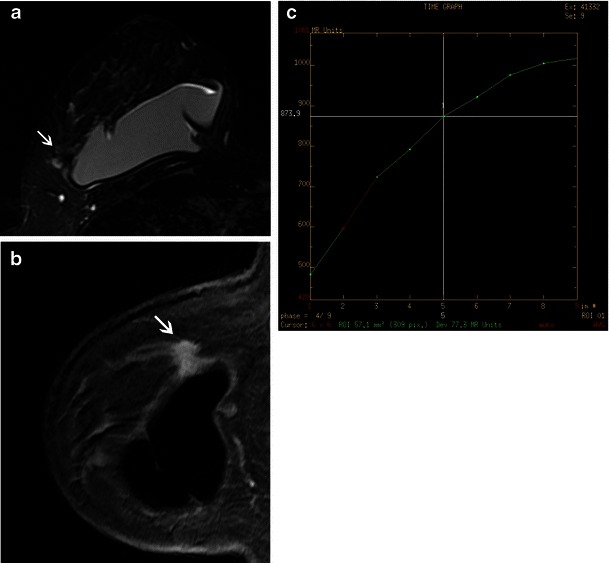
A 45-year-old woman with ILC. a and b MR images from right breast, respectively, T2 with fat suppression, sagittal reformation from T1 volumetric, post-contrast images, showing an irregular mass (*arrows*), with spiculated margins, seen adjacent to an intact breast implant. After IV contrast, there is a uniform, progressive enhancement, until delayed phases, typical of invasive lobular neoplasia. (c) Kinetic curve type 1
